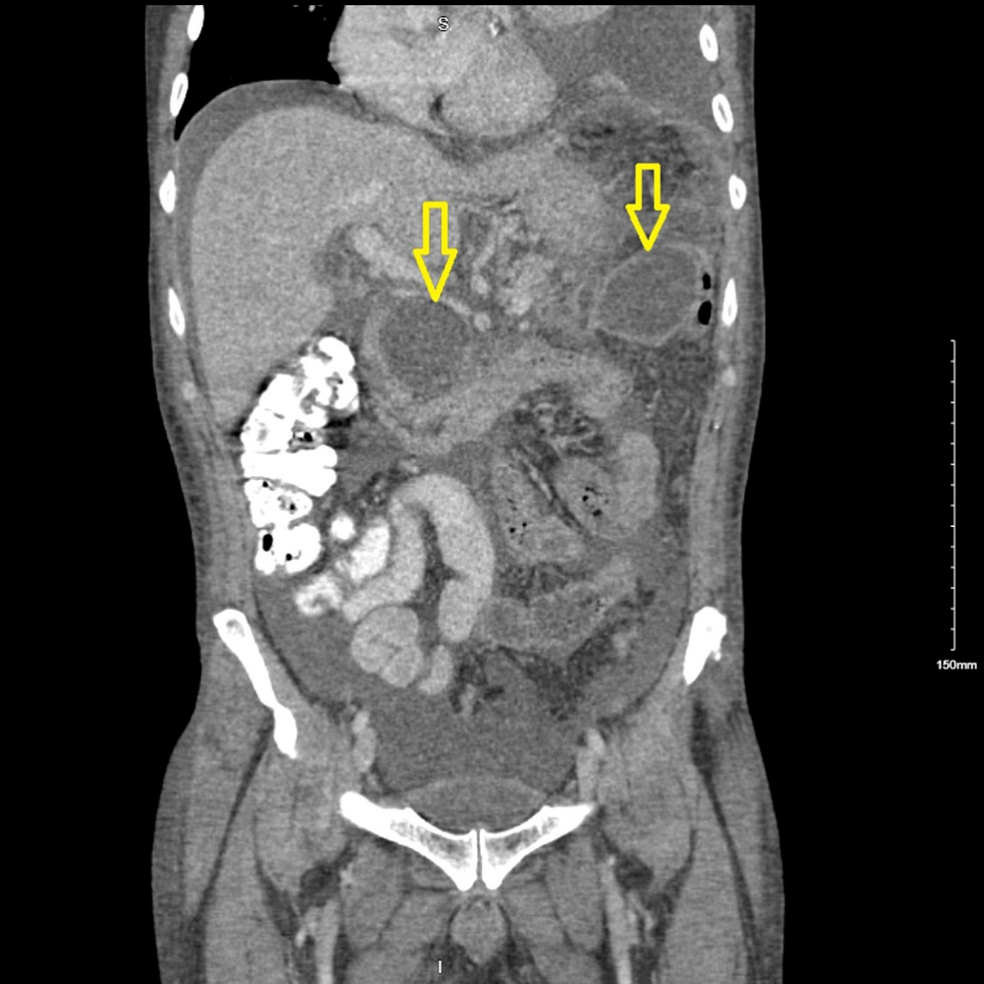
Coronal section of abdomen CT demonstrating ascites and pancreatic pseudocysts in the head and tail of pancreas, indicated with arrow marks.
